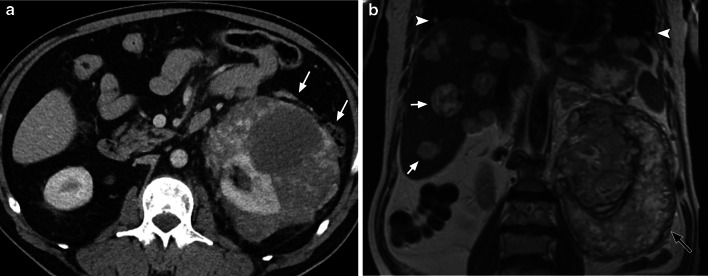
74-year-old man with angiosarcoma of the left kidney. a Axial contrast-enhanced CT image shows a large heterogeneously enhancing mass surrounding the left kidney occupying much of the peri-renal space displacing the adjacent mesenteric fat and bowel loops (arrows). There are numerous areas of low attenuation within the mass in keeping with necrosis. b Coronal T2-weighted image shows a large mass within the left peri-renal space (black arrow) consisting of predominantly high signal intensity, with low signal intensity striations within the mass. There are multiple metastatic deposits in the liver (white arrows) and lungs (arrowheads)
